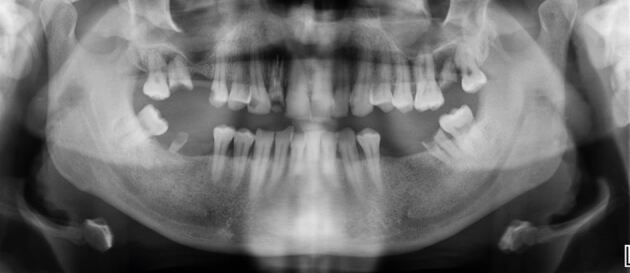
Radiograph of dentition of Case 3
